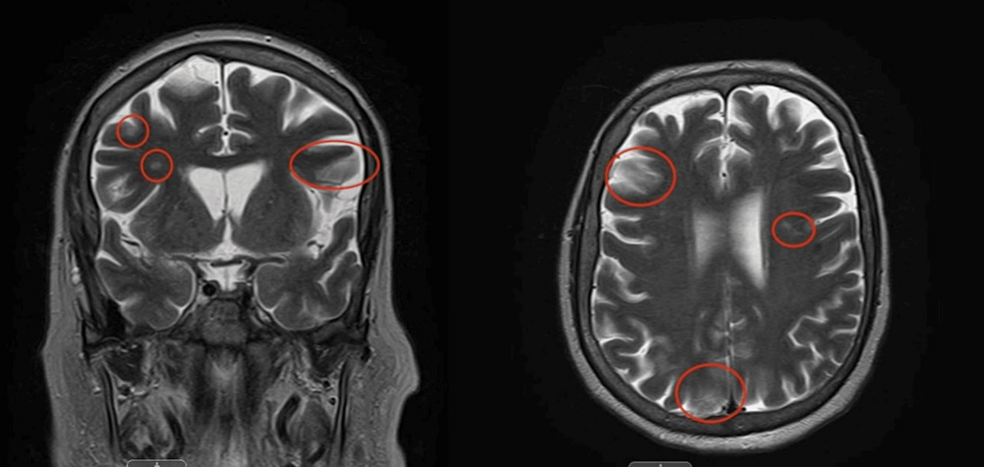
Coronal and Axial T2-weighted MRI showing scatted subcortical and ventricular white matter hyper-intensities. These may represent chronic small vessel ischemic disease or reversible encephalopathy and may be seen after seizures and metabolic disturbances.
